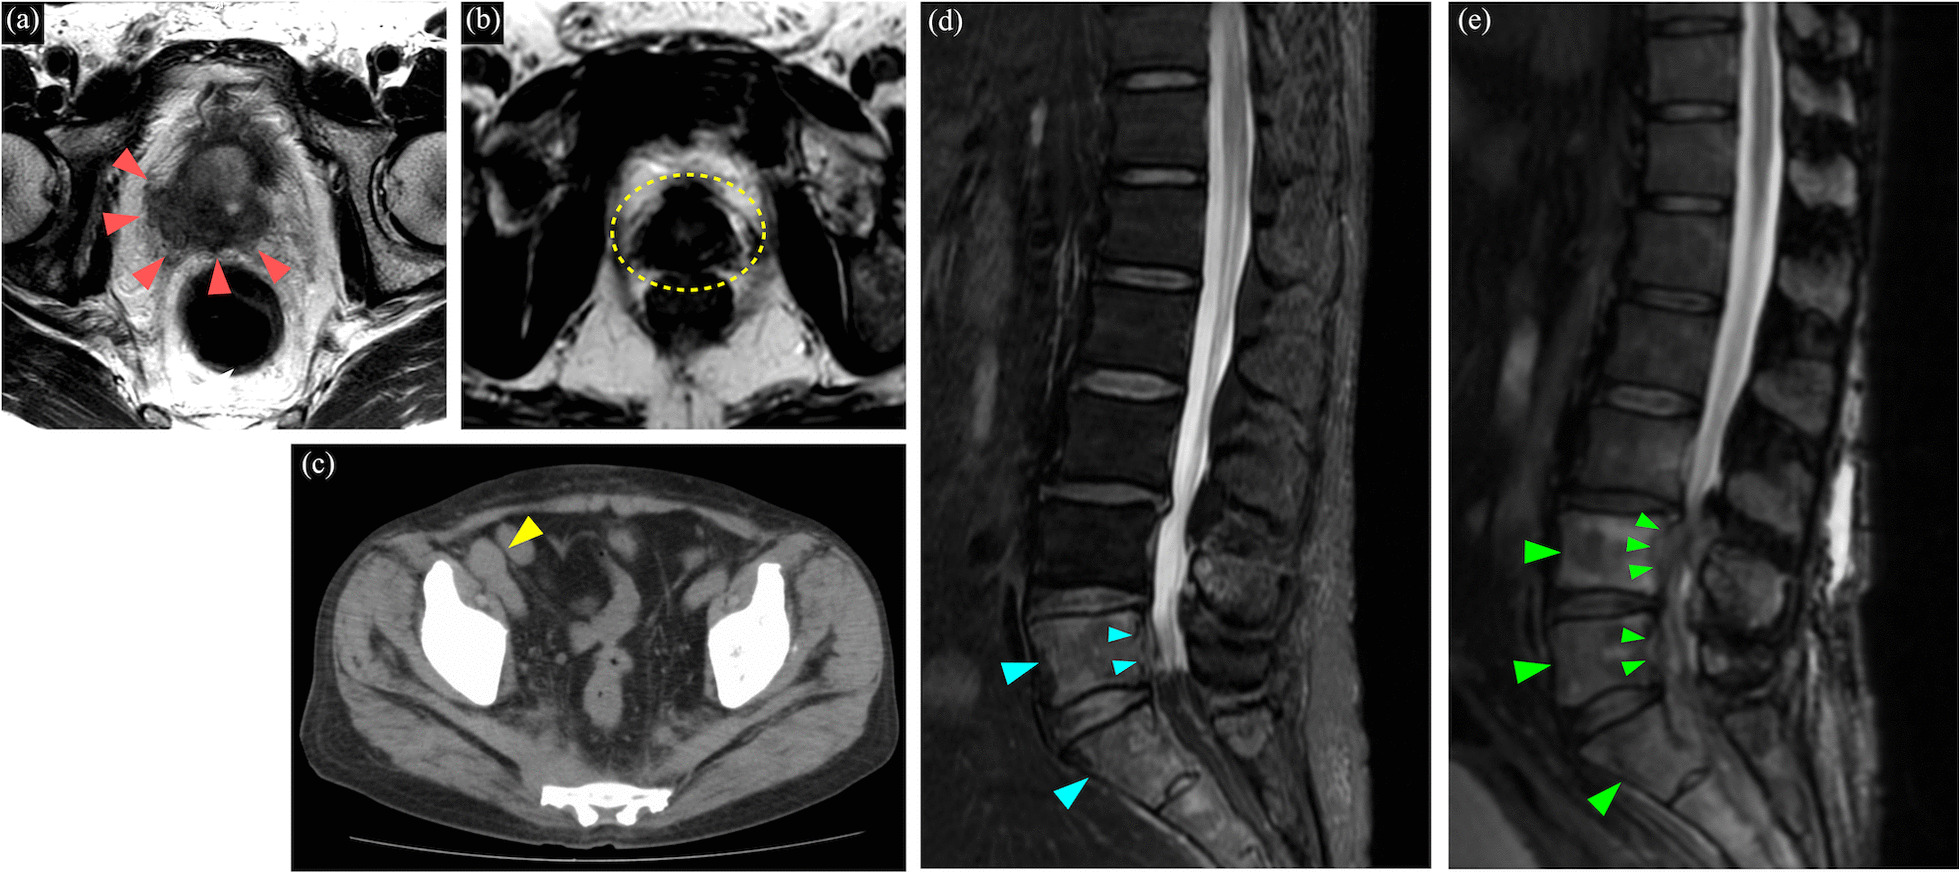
Primary and metastatic lesions during the treatment course. a T2WI of MRI showed a prostate tumor invading the right seminal vesicle at the primary diagnosis. b MRI showed that the primary lesion had shrunk after androgen deprivation therapy (ADT) and cabazitaxel (CBZ) therapy. The second biopsy revealed total elimination of the variable tumor. c T1WI of MRI with Gd enhancement showed the enlarged right inguinal lymph node, which was biopsied for whole-exome analysis and underwent WGD. d T2WI of lumbar MRI performed after CBZ showed diffuse metastases in L5/S1 vertebrae. The metastatic tumor in L5 was reaching toward the epidural space. e Lumbar MRI performed 2 years later showed the spreading of metastases to L4 vertebrae and spinal cord compression at the level of L4/L5, causing leg paralysis
